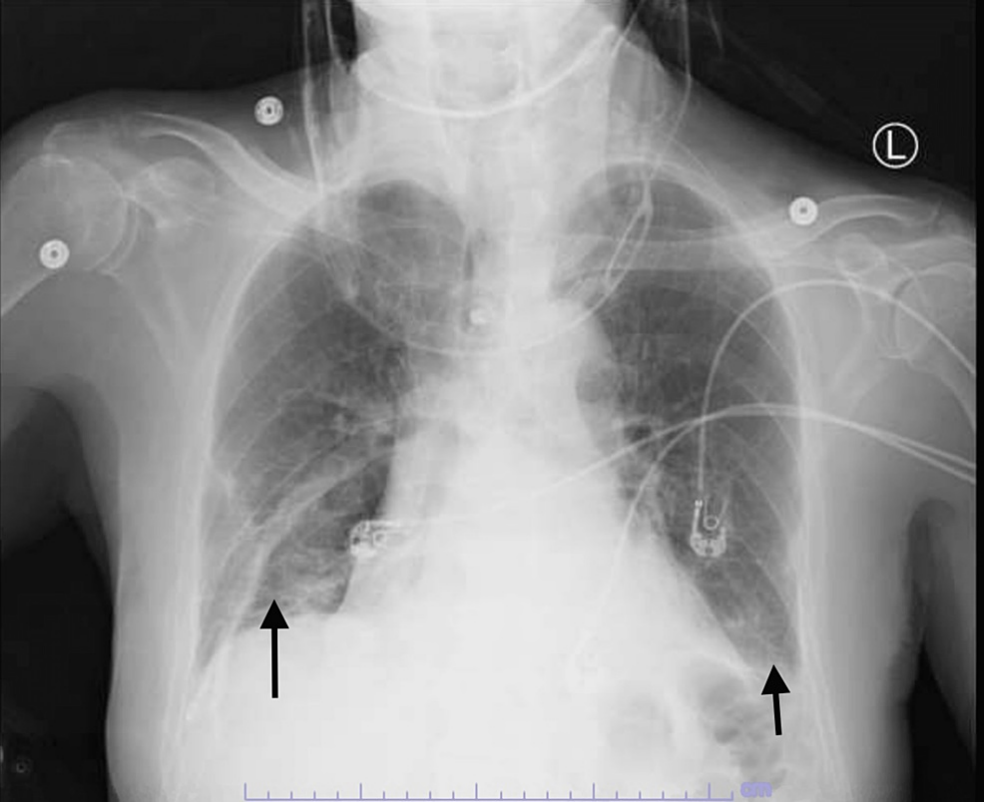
Chest X-Ray A chest x-ray showing evidence of bilateral basal opacities suggestive of COVID-19 pneumonia in the setting of acute hypodermic respiratory failure (arrows).
